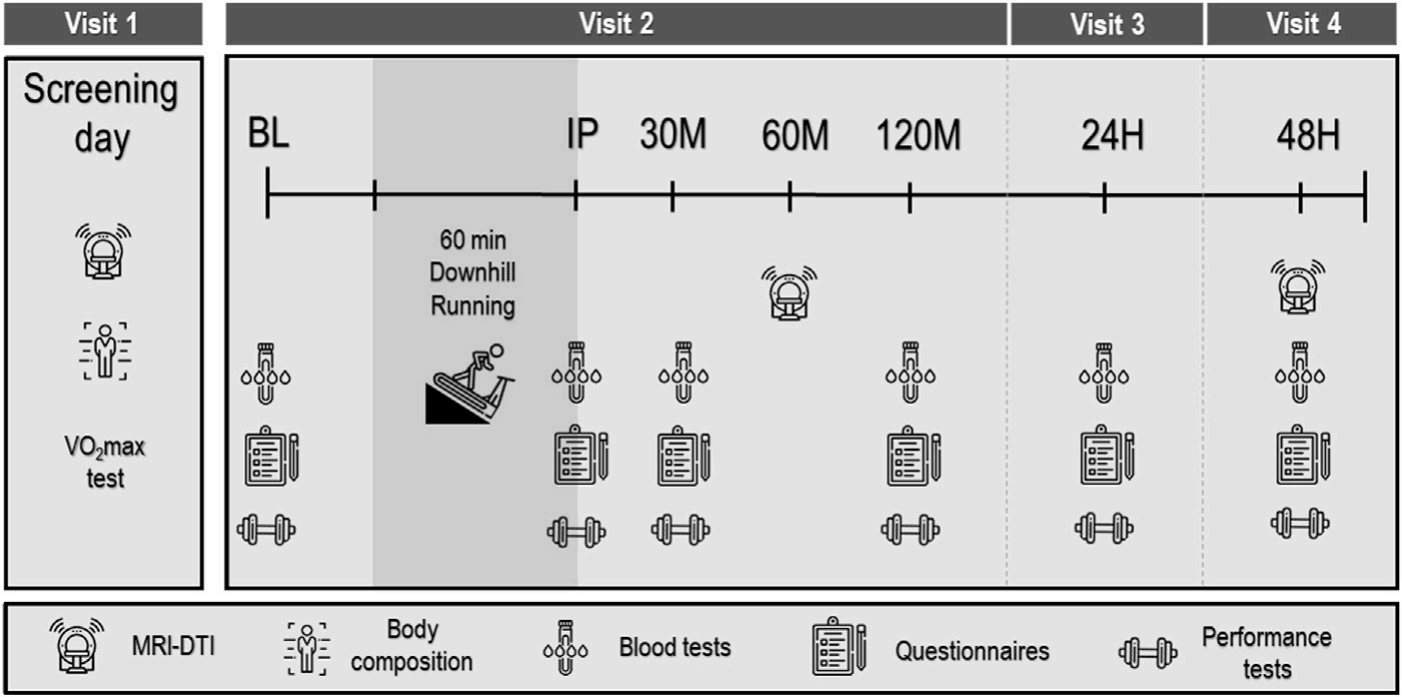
Experimental protocol. Baseline (BL), immediately post (IP), 30 min (30 M), 120 min (120 M), 24 h (24H) and 48 h (48H) post downhill running protocol. VO2max: maximal O2 consumption; MRI-DTI: magnetic resonance imaging diffusion tensor imaging.
